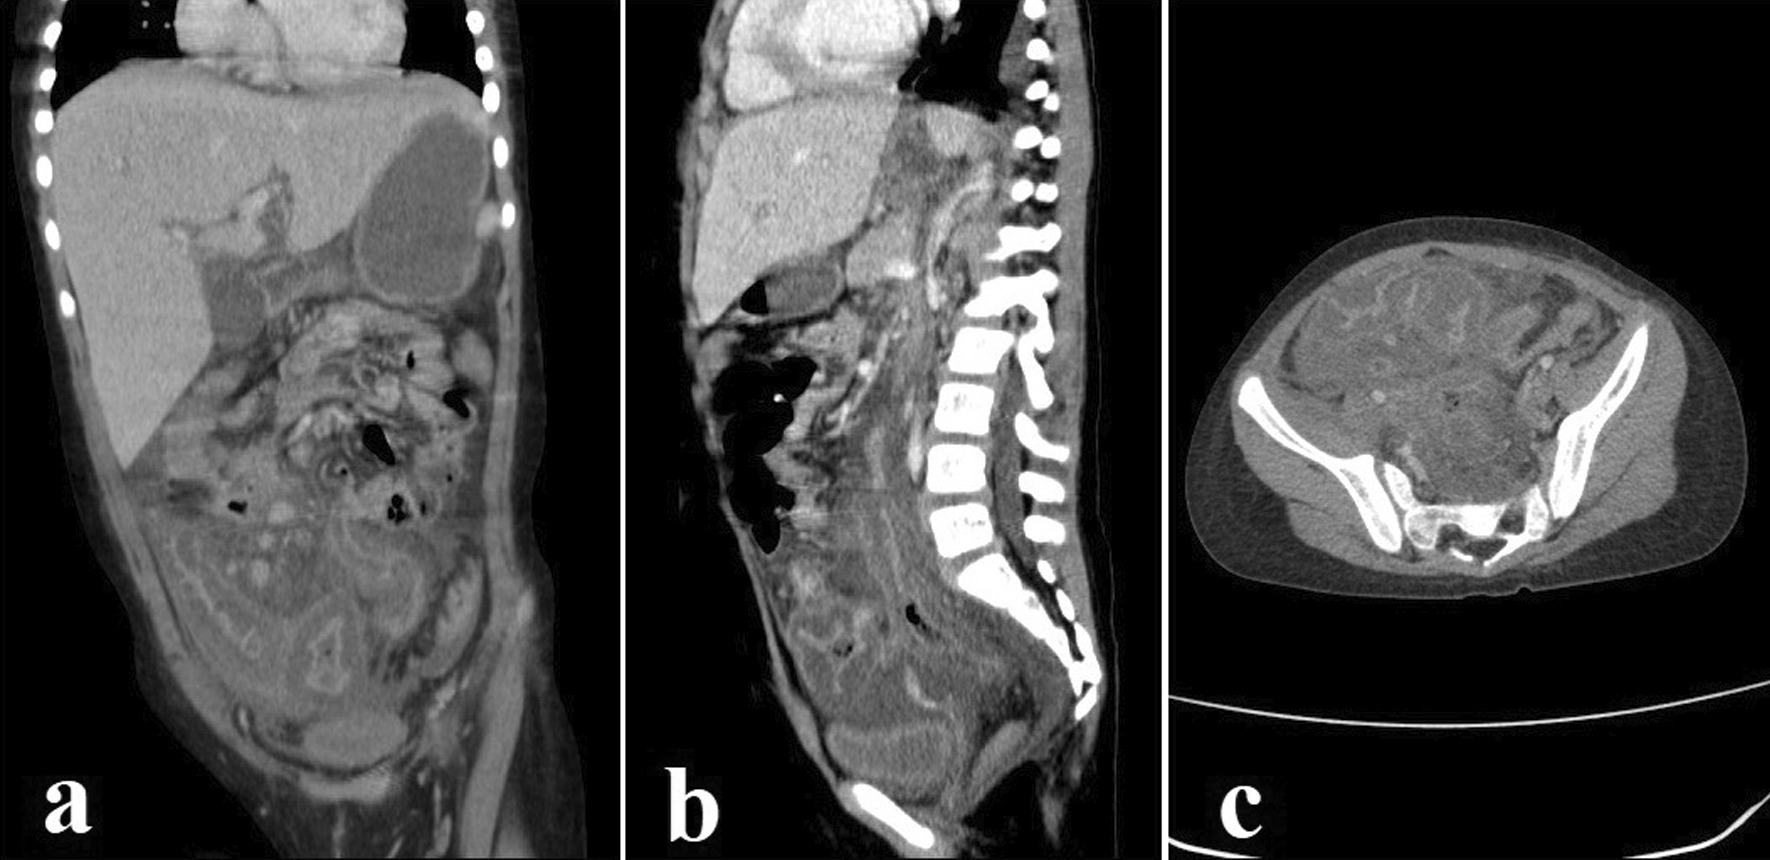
a–c Coronal, sagittal, and axial reconstruction images through the abdomen and pelvic spiral CT scan after intravenous and oral contrast administration demonstrate mild amount of ascitis in abdominopelvic cavity, significant wall edema of cecum and ascending colon with severe adjacent fat stranding and edema. Also, significant wall edema of sigmoid colon and multiple sub-centimeter mesenteric lymph nodes is seen. Significant haziness of mesenteric fat and peritoneum is seen in favor of peritonitis
